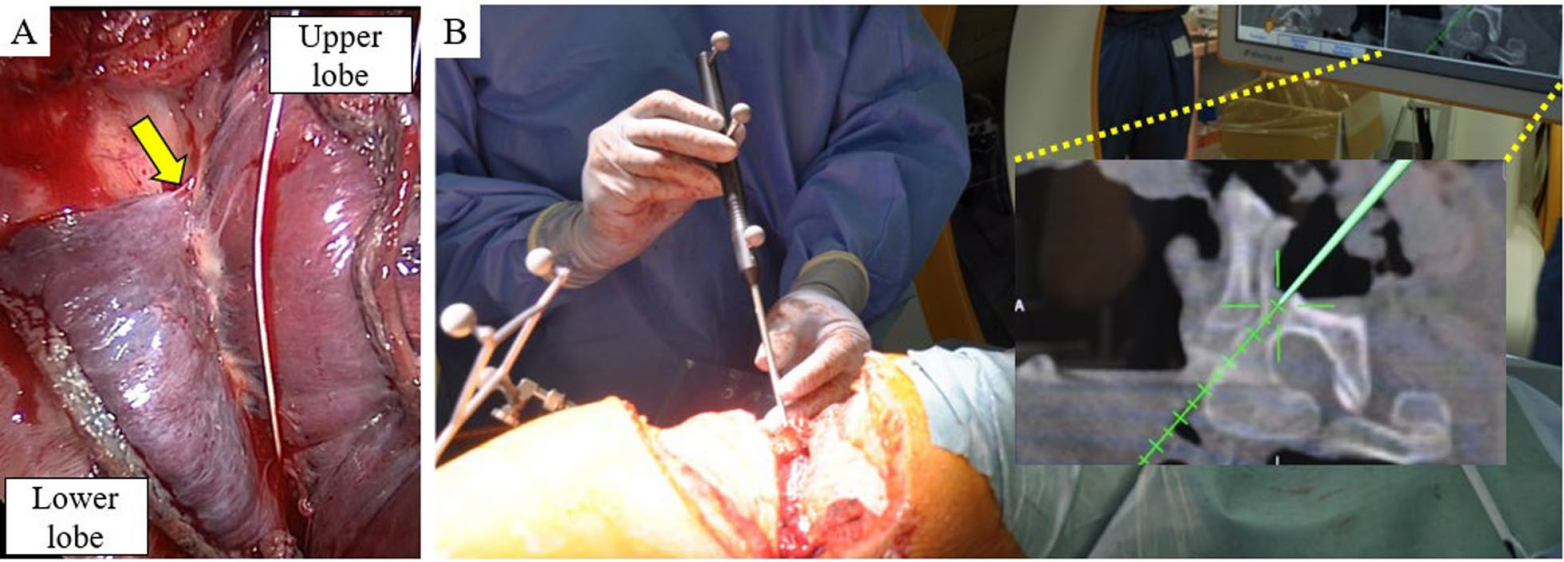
Intraoperative findings in case 1. A Intraoperative findings showed that the tumor was primarily located in the chest wall and involved both upper and lower lobes (arrow). B A CT-based navigation system was used for vertebrectomy, and the monitor shows the planned cutting line in green
